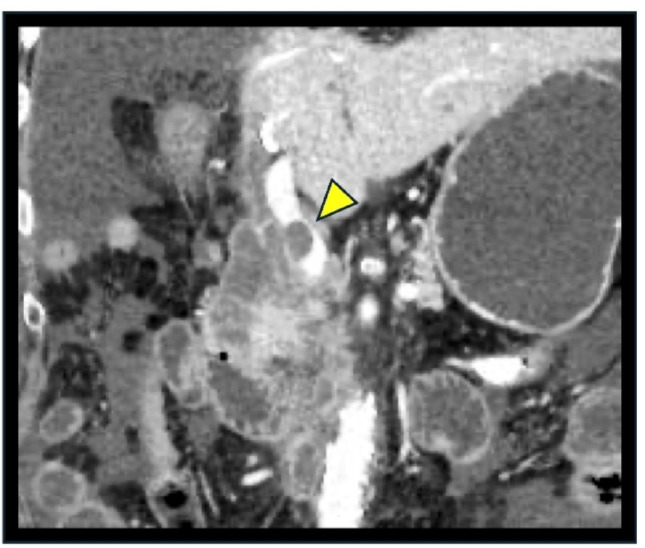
Contrast-enhanced computed tomography reveals massive ascites and portal vein thrombosis on postoperative day 6 (arrowhead)
